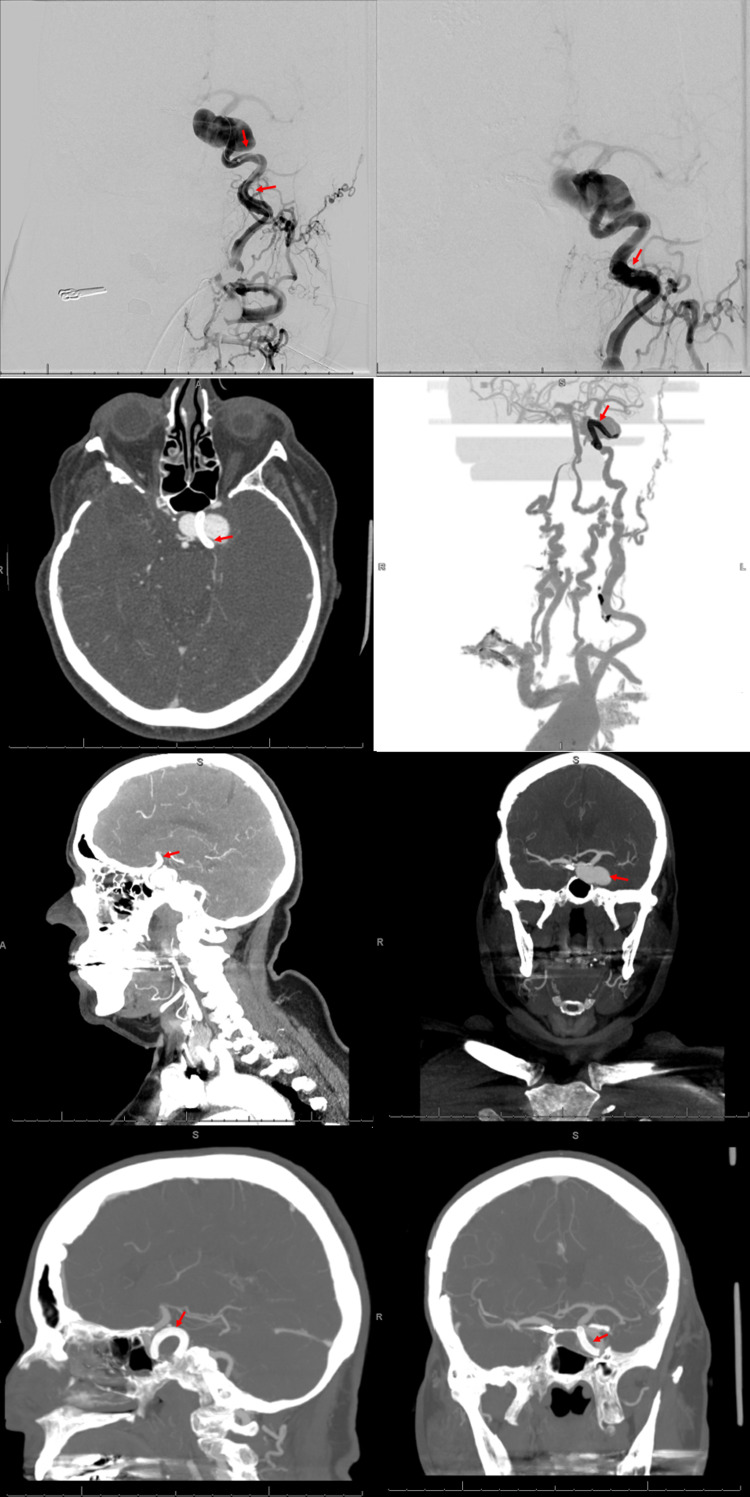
PED placement of right cavernous ICA aneurysm Top row, left: Digital subtraction angiography (DSA) demonstrating the use of a quad axial technique with the long sheath, flow gate guide catheter, and phenom plus in the distal cervical internal carotid artery. The aneurysm was then approached using a Synchro microguidewire. The ophthalmic segment and supraclinoid segment of the internal carotid artery were catheterized. The Asahi 18 microguidewire was used to provide support for this catheter to be placed near the M1 carotid terminus as indicated by the red arrows. Seen as well is the previous surgical clip placement of the right ICA. Top row, right: A 4.25 × 30 mm PED was deployed successfully across the neck of the aneurysm with the tail end of the stent noted by the red arrow. Second row: Postoperative follow-up axial non-contrast CT of the head and with reconstruction demonstrating PED placement across the neck of the supraclinoid aneurysm (red arrows). Third row: Initial preoperative CT of the head with contrast in sagittal and coronal views demonstrating an approximately 27 × 15 × 15 mm cavernous ICA aneurysm without evidence of occlusion or stenosis (red arrows). Bottom row: Six-month follow-up CT angiography in sagittal and coronal views demonstrating absent filling of left cavernous ICA aneurysm with patent stent placement indicated by the red arrows.
